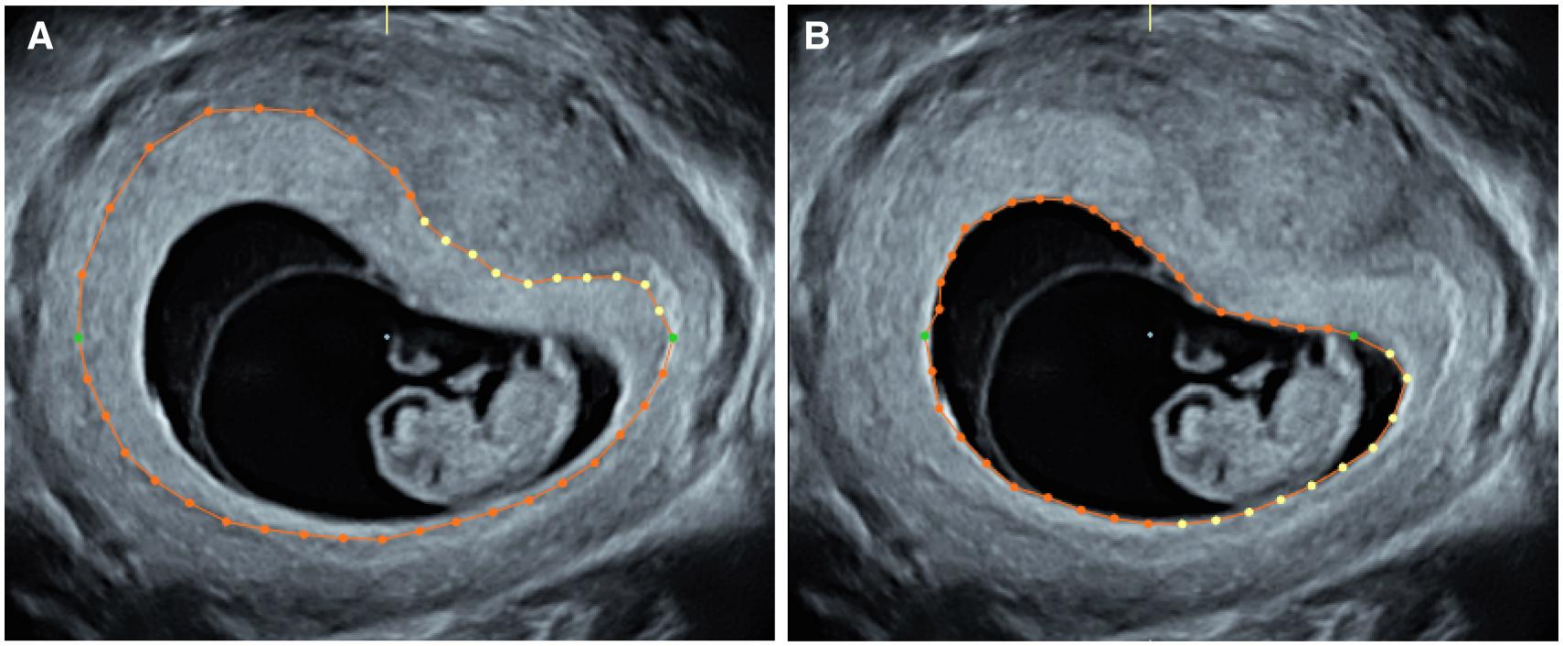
Measuring placental volume (PV) using 3-dimensional ultrasound volumes. The PV is calculated by the total pregnancy volume (A) minus the volume of the gestational sac (B).
